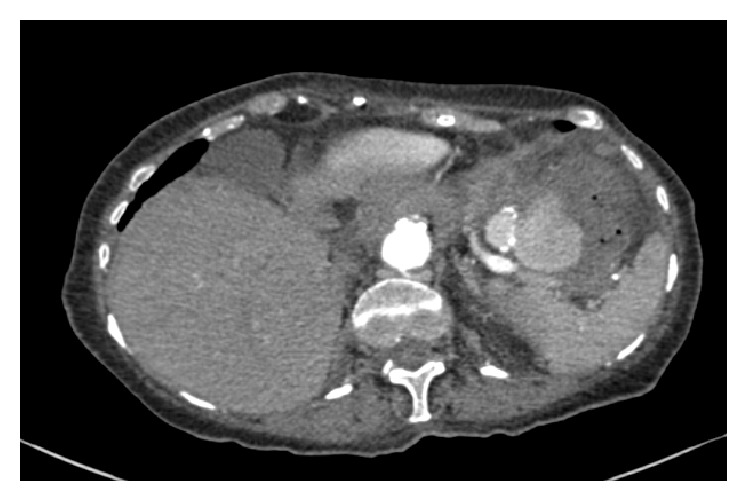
CT angiography axial plane demonstrating splenic artery pseudoaneurysm.
